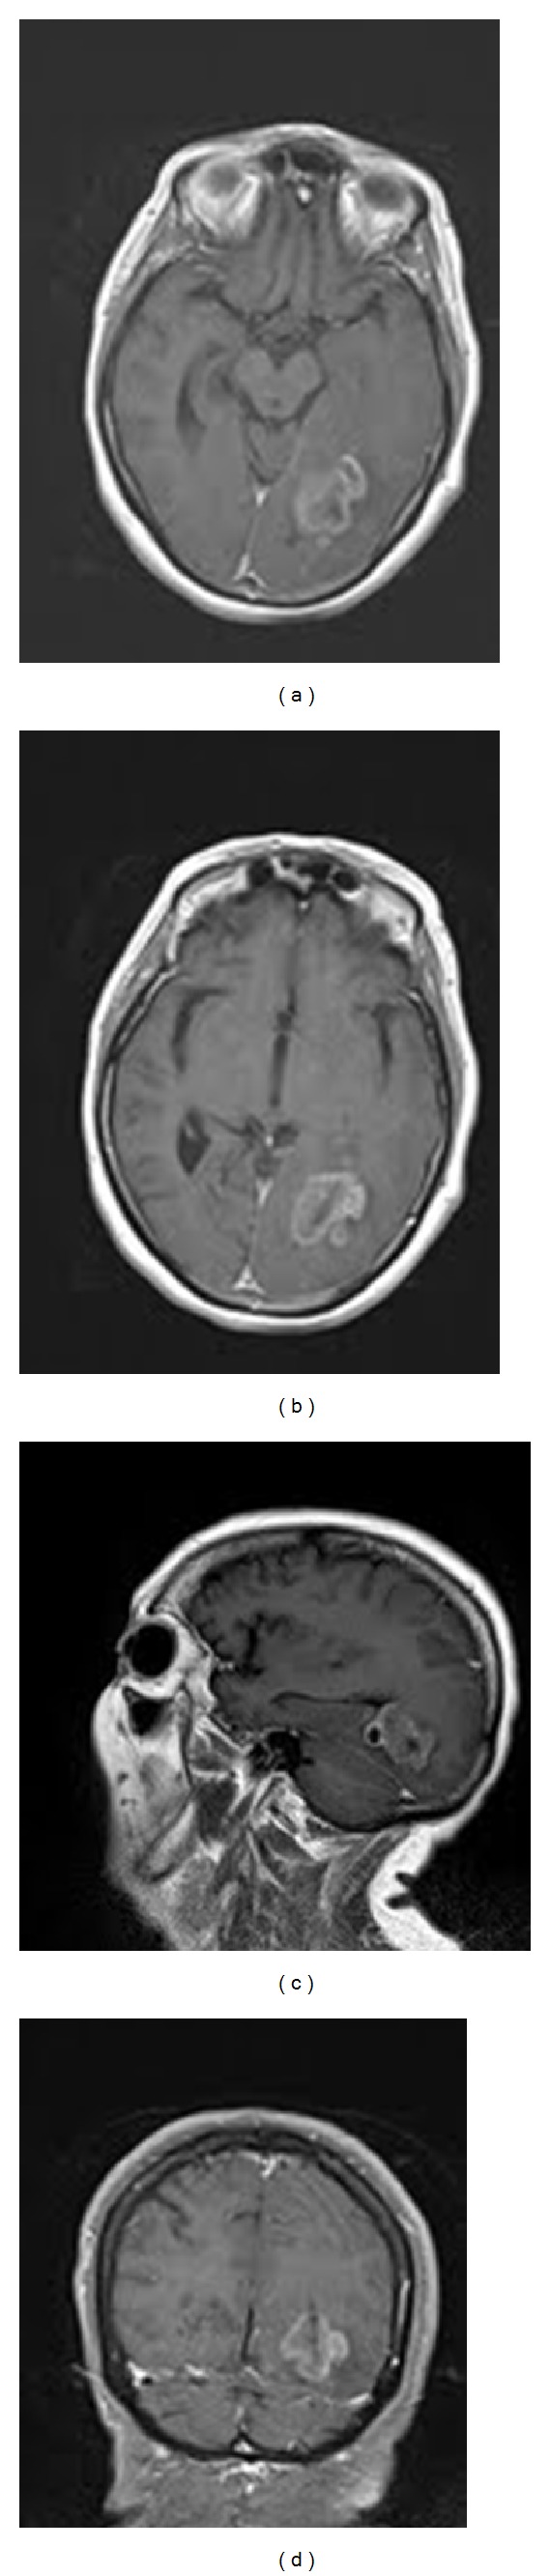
Initial brain MRI. (a), (b) Axial T1-weighted image with gadolinium, showing the enhancing intra- and periventricular left occipitotemporal mass. There is minimal mass effect. In (b) there is a suggestion of a “daughter cyst” lateral to the main lesion. (c) Sagittal T1-weighted image, with gadolinium, showing the lesion mostly within the occipital horn of the lateral ventricle. (d) Coronal T1-weighted image with gadolinium. The abnormal tissue involves the parenchyma around the ventricle as well as within the lumen.
